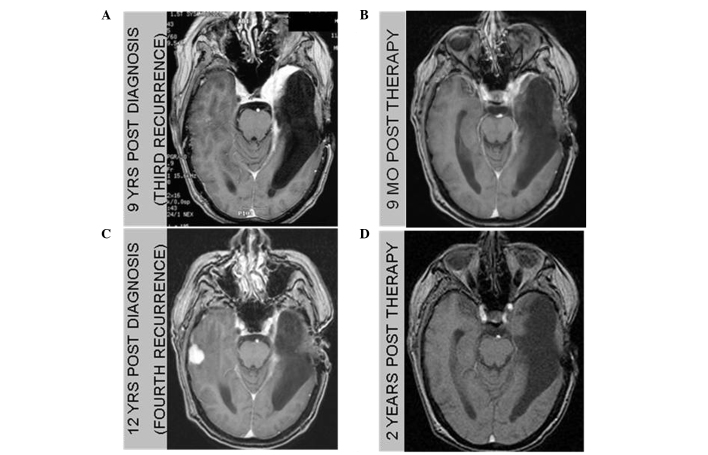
Axial MRI T1 with contrast images. (A) Third recurrence, nine years following diagnosis, of an enhancing lesion in the anterior temporal fossa involving the left optic nerve. (B) Resolution of the lesion following 9 months of steroid therapy. (C) Fourth recurrence, 12 years following diagnosis, consisting of a new contrast-enhancing lesion in the contralateral temporal lobe. (D) Two-year follow-up showing complete sustained resolution of the lesion following seven months of steroid therapy.
